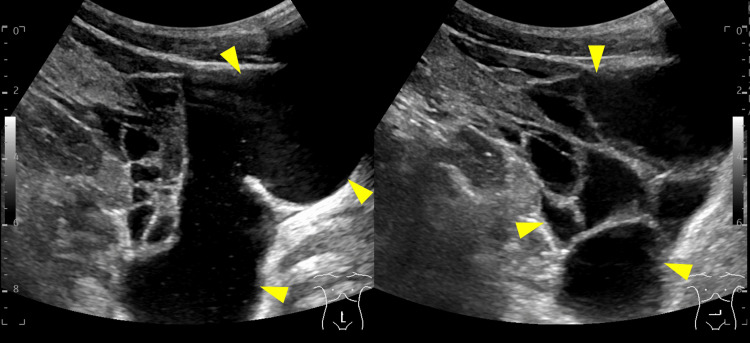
Transcutaneous abdominal echography The fluidlike space in computed tomography (CT) comprised multiple cystic lesions (arrowheads)
